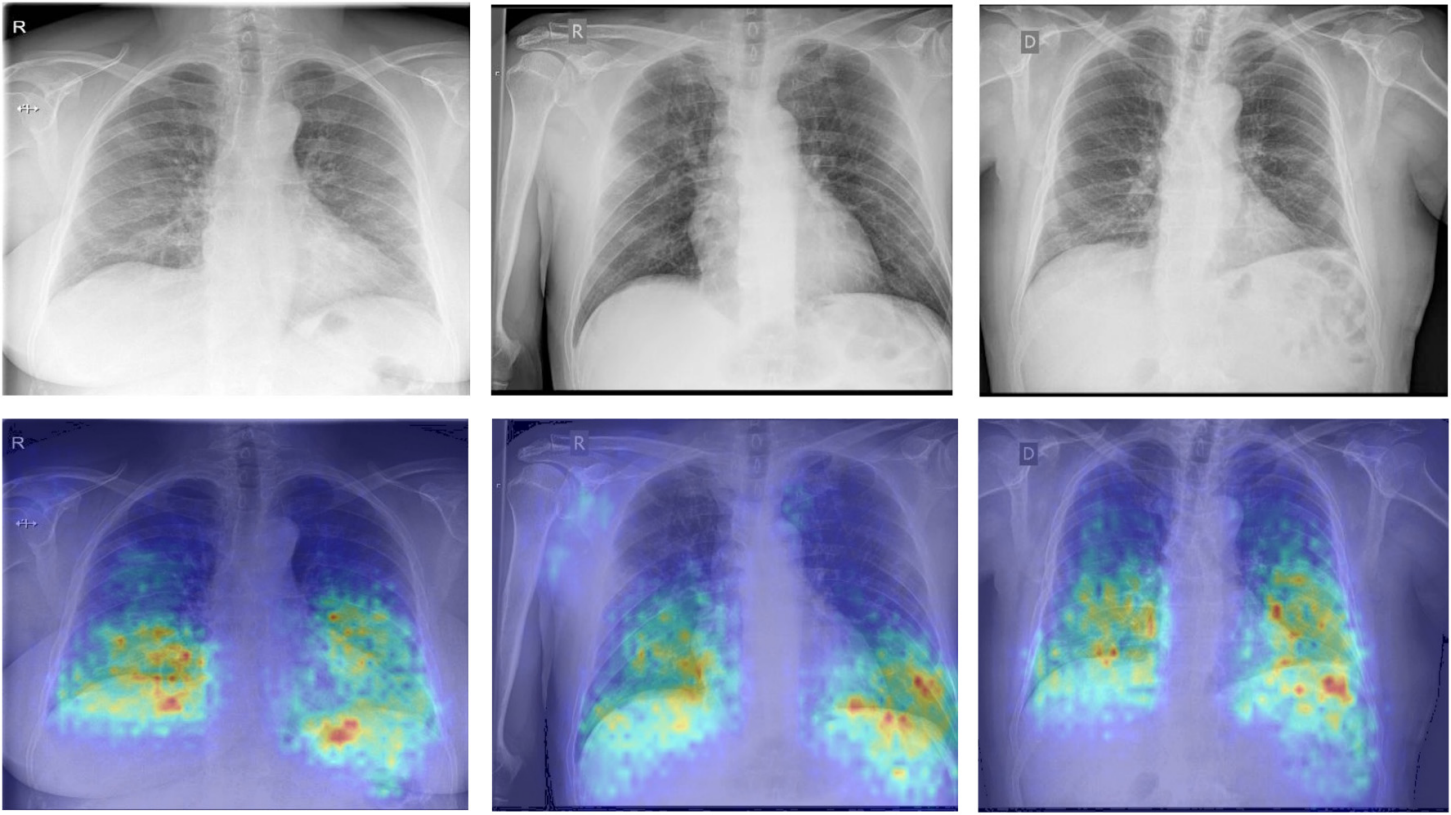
Representative activation maps of input chest X-ray images using GradCAM, where the redder tones represent the activations with a higher intensity and the darker blue tones represent the activations with a smaller intensity.
